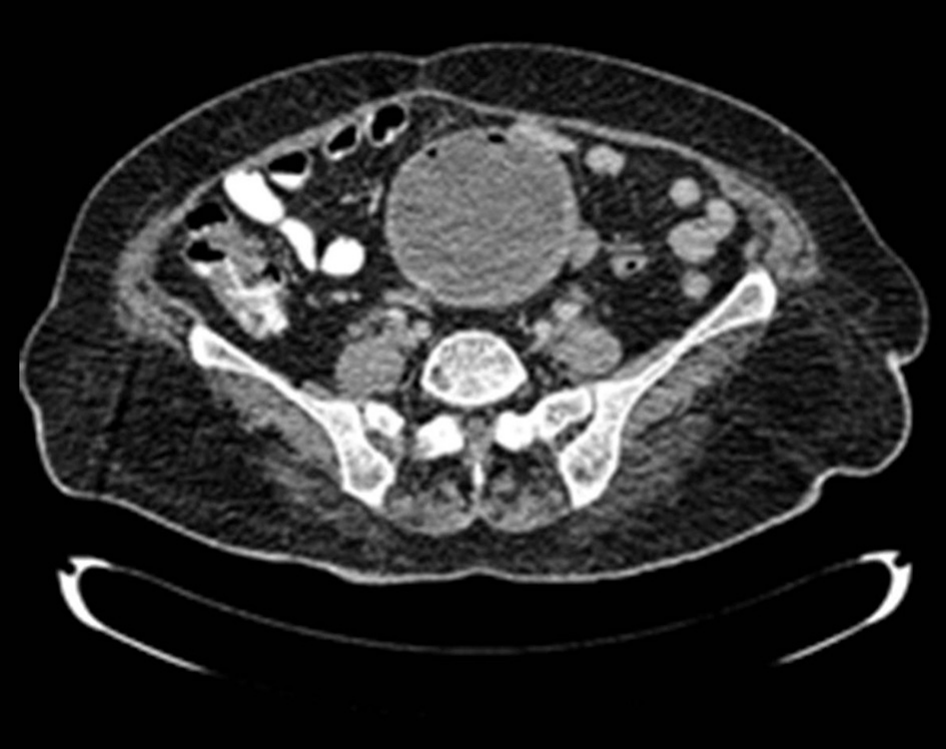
CT-Scan: A round mass, cystic lesion and focal calcification at its periphery, probably ovarian cyst adenoma.
